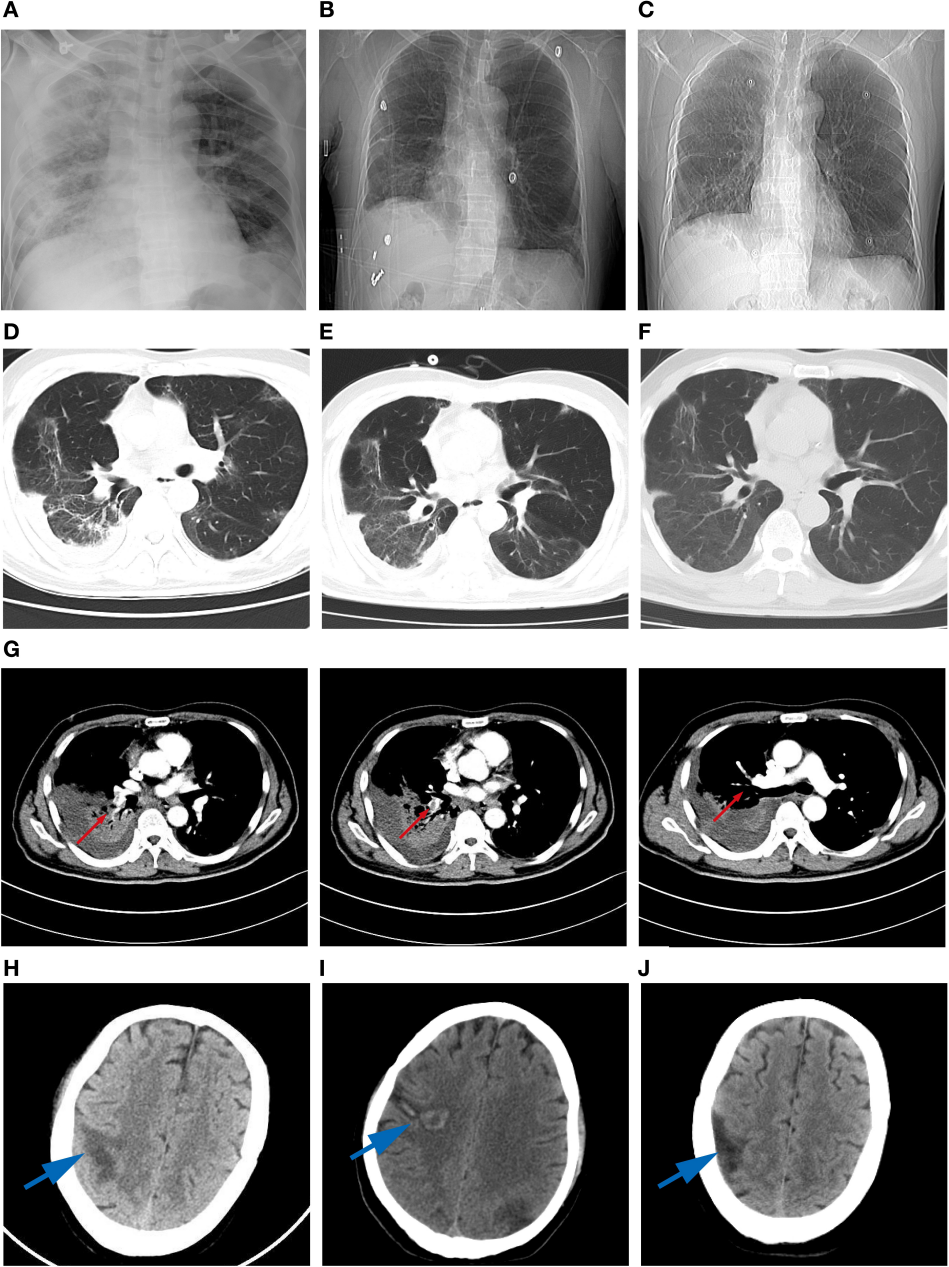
High-resolution computed tomography images during the disease course. (A) chest X-ray image revealing ground-glass opacities (GGO) in the both lobes on day 13 of hospitalization. (B) GGO in the both lobes on day 36. (C) Thickened lung markings in the right lobe on day 44. (D) Multiple-ground-glass opacification in the basal segment of lobes on day 17. (E) GGO in both lower lobes on day 27. (F) GGO in the middle lobe of the right lung on day 44. (G) Filling defect in the trunk and branches of the right inferior pulmonary artery (red arrow). (H) Slightly low-density shadows in the right parietal lobe and the left temporal lobe on day 17 of hospitalization (blue arrow). (I) Hemorrhagic infarction in the right parietal lobe on day 27 (blue arrow). (J) Low-density shadows in the right parietal lobe on day 44 (blue arrow).
